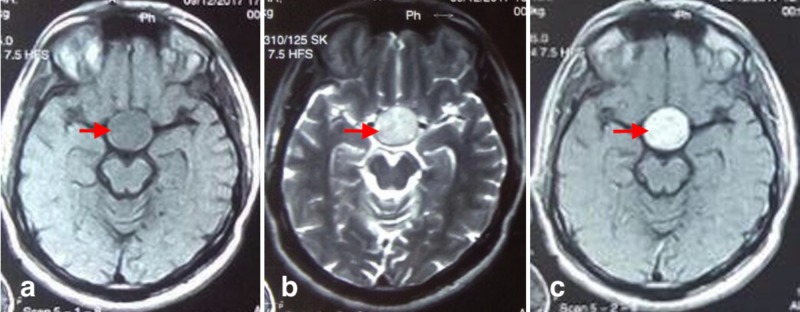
(a) T1-weighted axial image showing hypointense sellar lesion with parasellar extension. (b) T2-weighted axial image showing hyperintense sellar lesion with parasellar extension. (c) T1-weighted image with gadolinium contrast showing diffuse contrast enhancement of the lesion.
